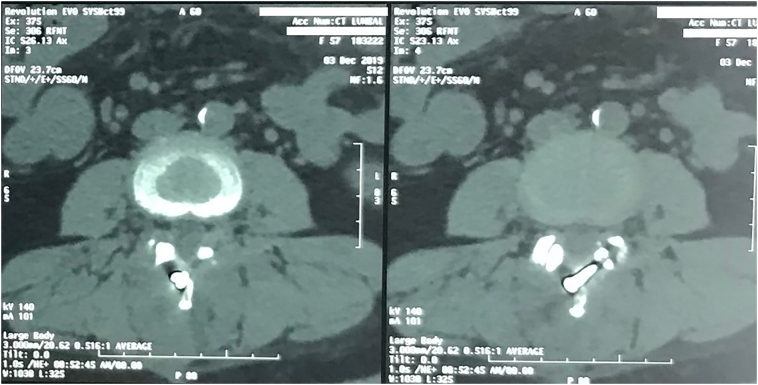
CT Scan of a 58-year-old female one year after surgery. There was a radiolucent halo, indicating peri-implant osteolysis around the screw.
